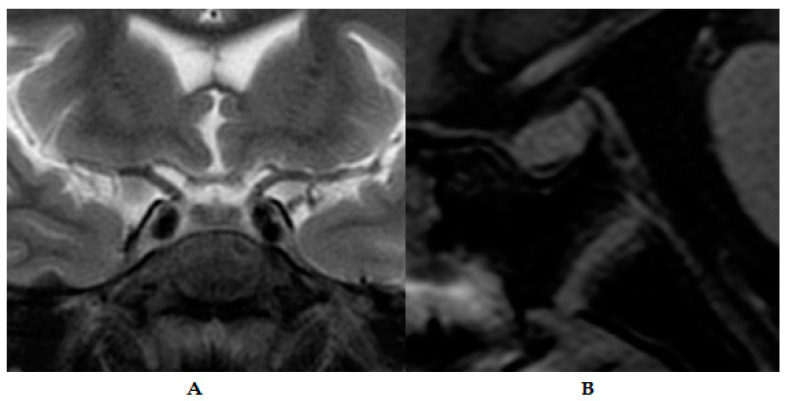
Contrasted magnetic resonance imaging (MRI) of pituitary gland. (A) Coronal view. (B) Sagittal view.
